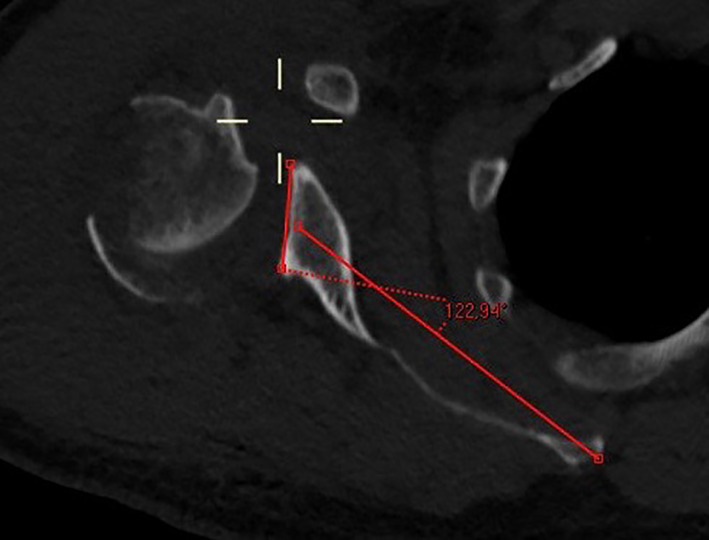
Axial CT slice in the scapular plane displaying glenoid retroversion of >30°, as often seen in dysplastic Walch C type glenoids. The greater tuberosity is detached from the humeral head in this four‐part proximal humeral fracture.
